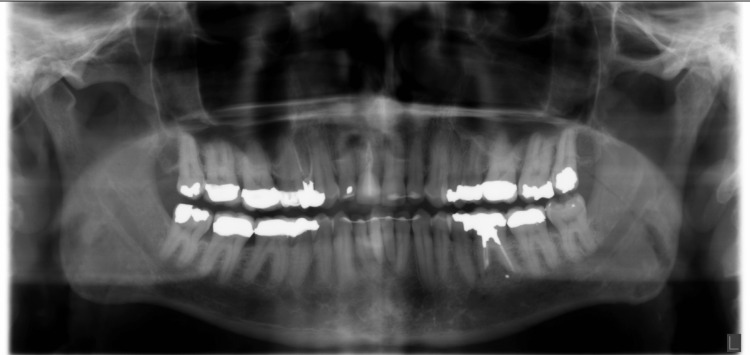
Panoramic radiograph: the three mental foramina are not visualized on the left side.
